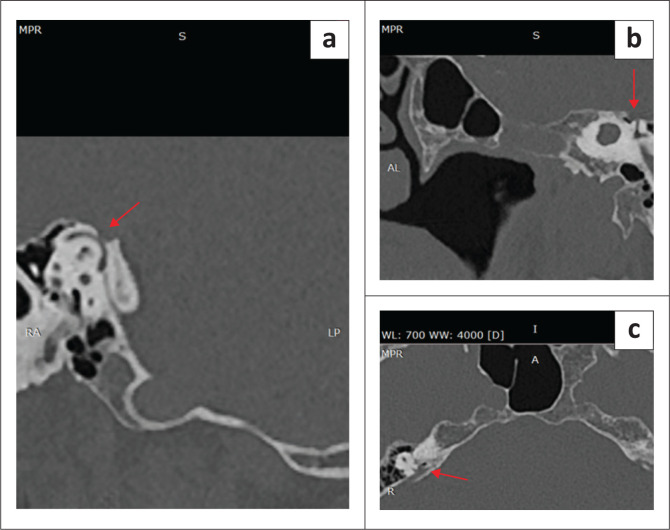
Superior semicircular canal dehiscence in a 35-year-old female patient. (a) Reformatted CT image in the Pöschl plane, clearly demonstrating the bony defect over the superior semicircular canal (red arrow). (b) High-resolution coronal reconstruction displaying the superior semicircular canal perpendicular to the long axis of the temporal pyramid (red arrow). (c) Stenvers plane reconstruction, oblique to the Pöschl plane and parallel to the long axis of the pyramid (red arrow).
